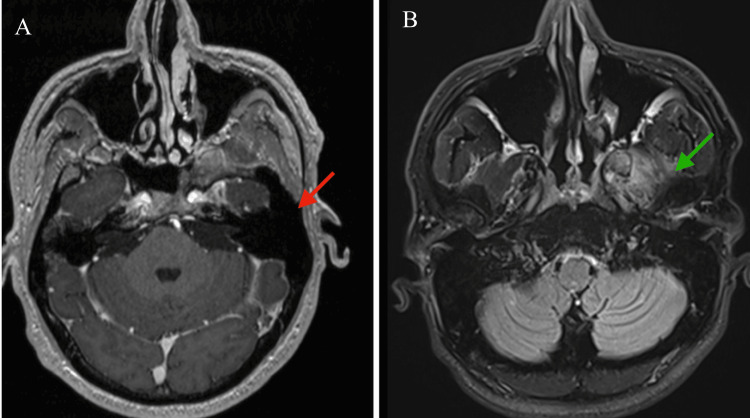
Axial contrast-enhanced MRI of the face demonstrating sustained complete remission of extranodal diffuse large B-cell lymphoma (A) T1-weighted post-contrast MRI (axial view) obtained at the same anatomic level demonstrating no residual or recurrent enhancing lesion (red arrow). (B) T1-weighted post-contrast MRI (axial view) obtained at the same anatomic level as the initial CT, demonstrating fibrosis with no residual or recurrent enhancing lesion (green arrow). No biopsy of the fibrotic-appearing area was performed.
